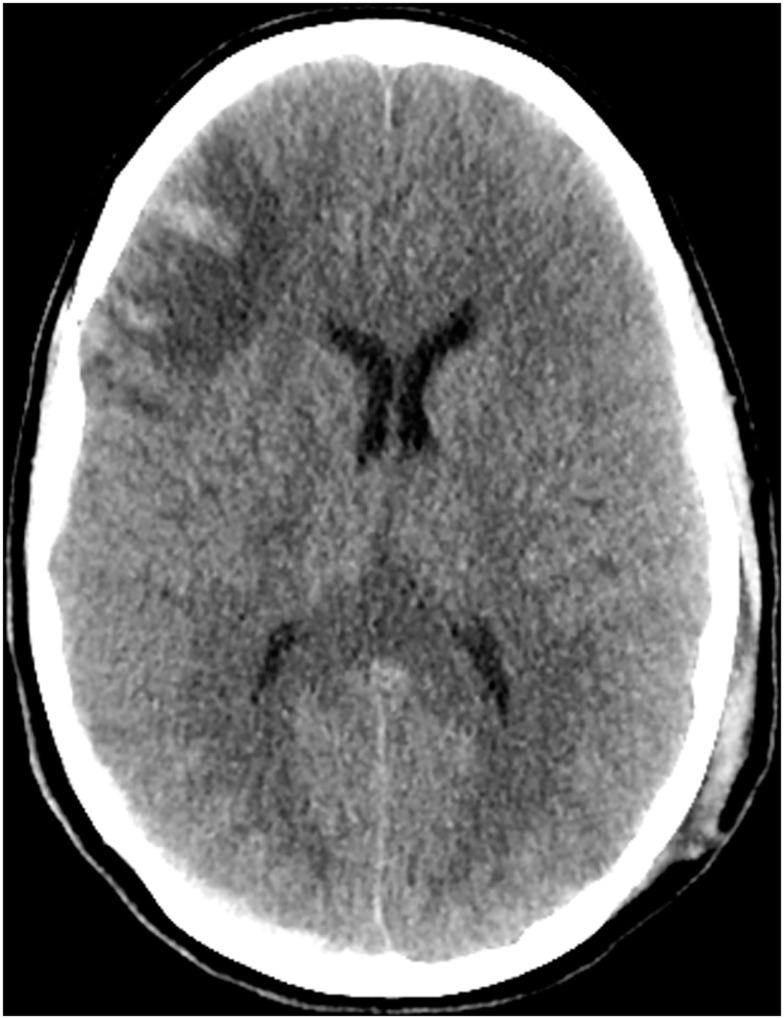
CT scan of the head on hospital day 11 demonstrates expected evolution of the previously noted cortical contusions, subdural hematomas, and changes of diffuse axonal injury. No new hemorrhage, mass effect, hydrocephalus, or CT radiographic evidence of acute ischemic injury. Stable diffuse cerebral swelling with partial effacement of the suprasellar cistern.
